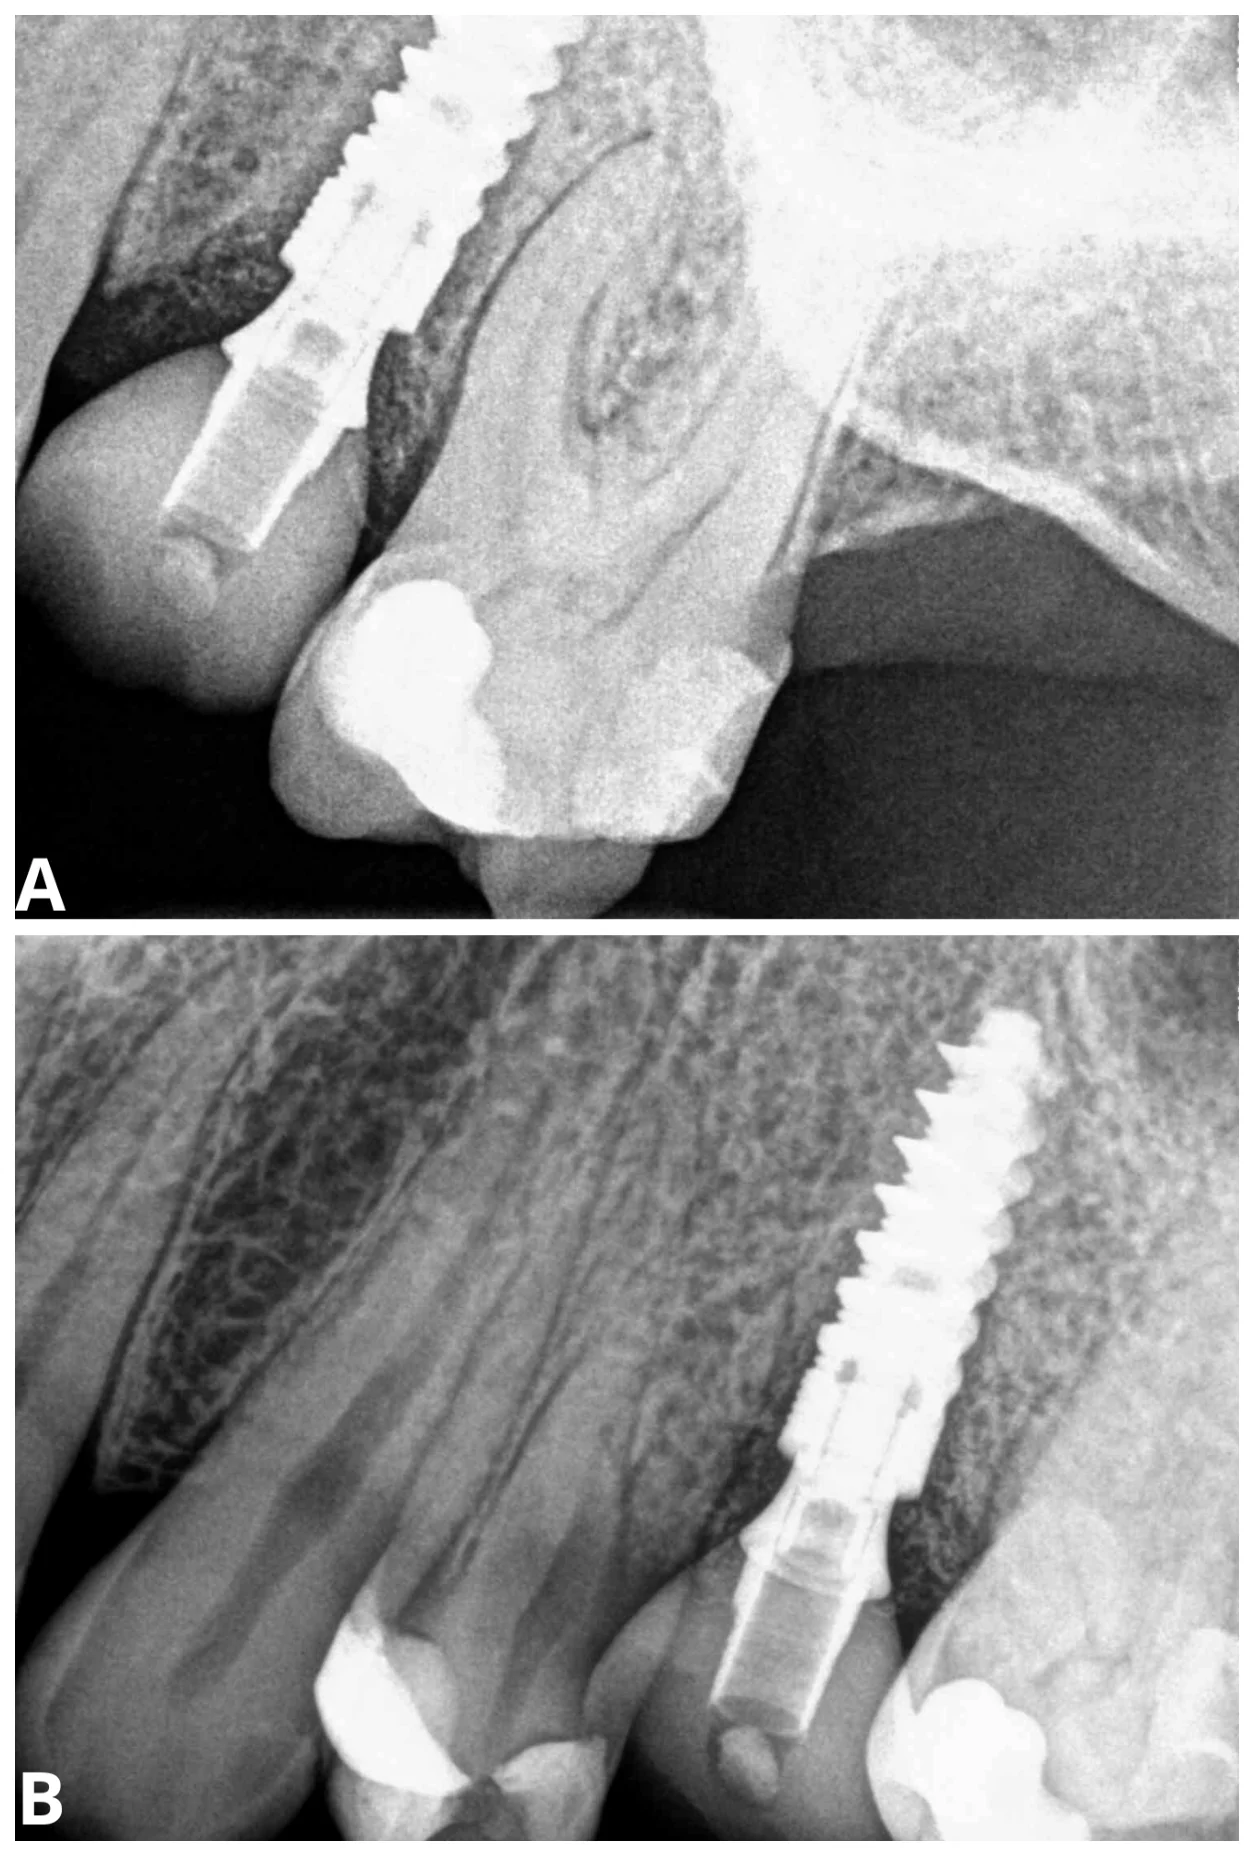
Radiographic follow-up: (A)—one month postoperatively; (B)—two months postoperatively.
